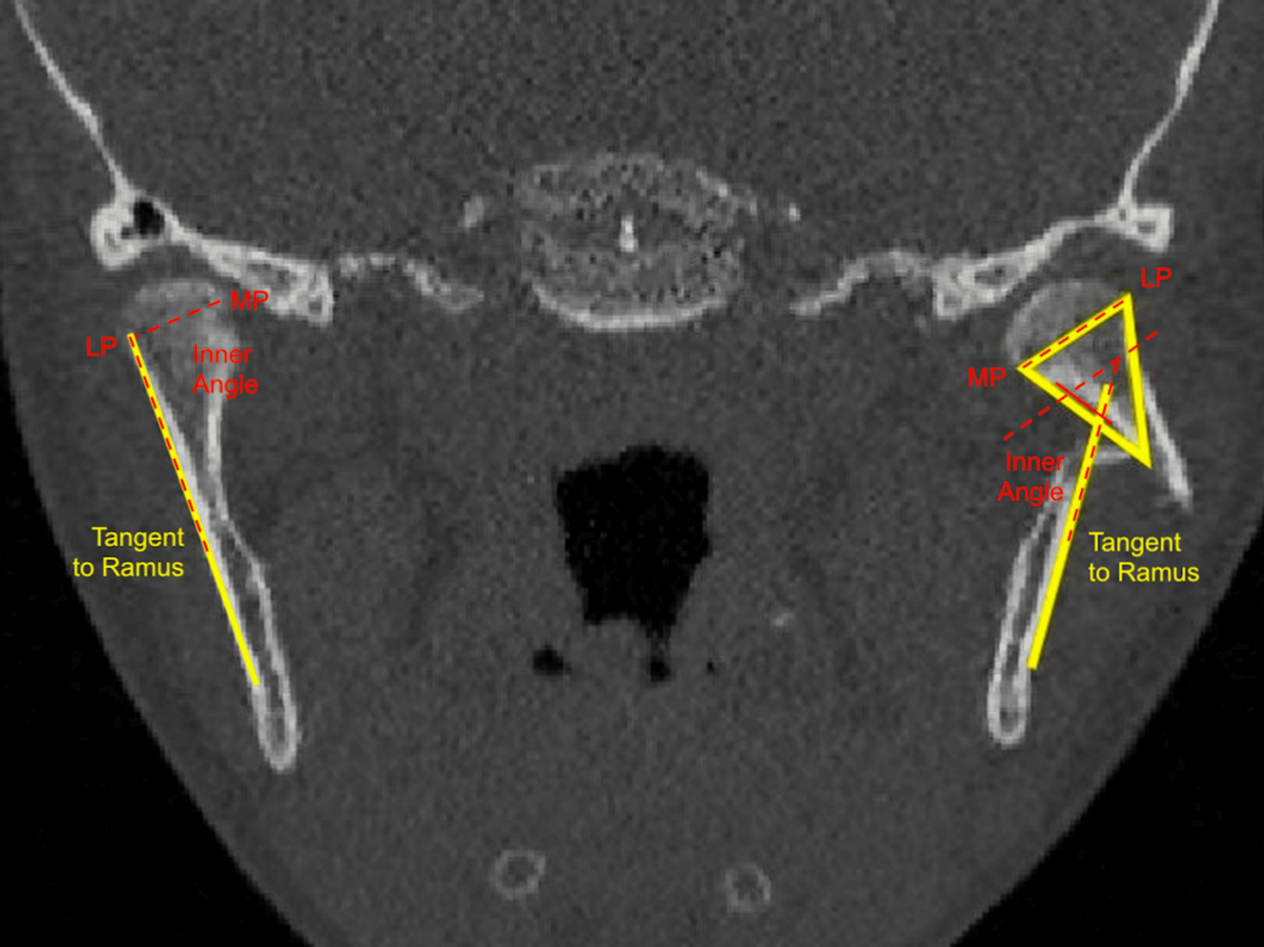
Condylar displacement is quantified on coronal CBCT image using the Vidhya et al. method A line is drawn between the medial and lateral poles of the condyle. Another line is drawn tangent to the ramus. The inner angle formed by the intersection of the two lines is calculated. The difference between the angles on the non-fractured and the fractured sides is used as a measure of coronal displacement. CBCT: cone beam computed tomography; LP: lateral pole; MP: medial pole
